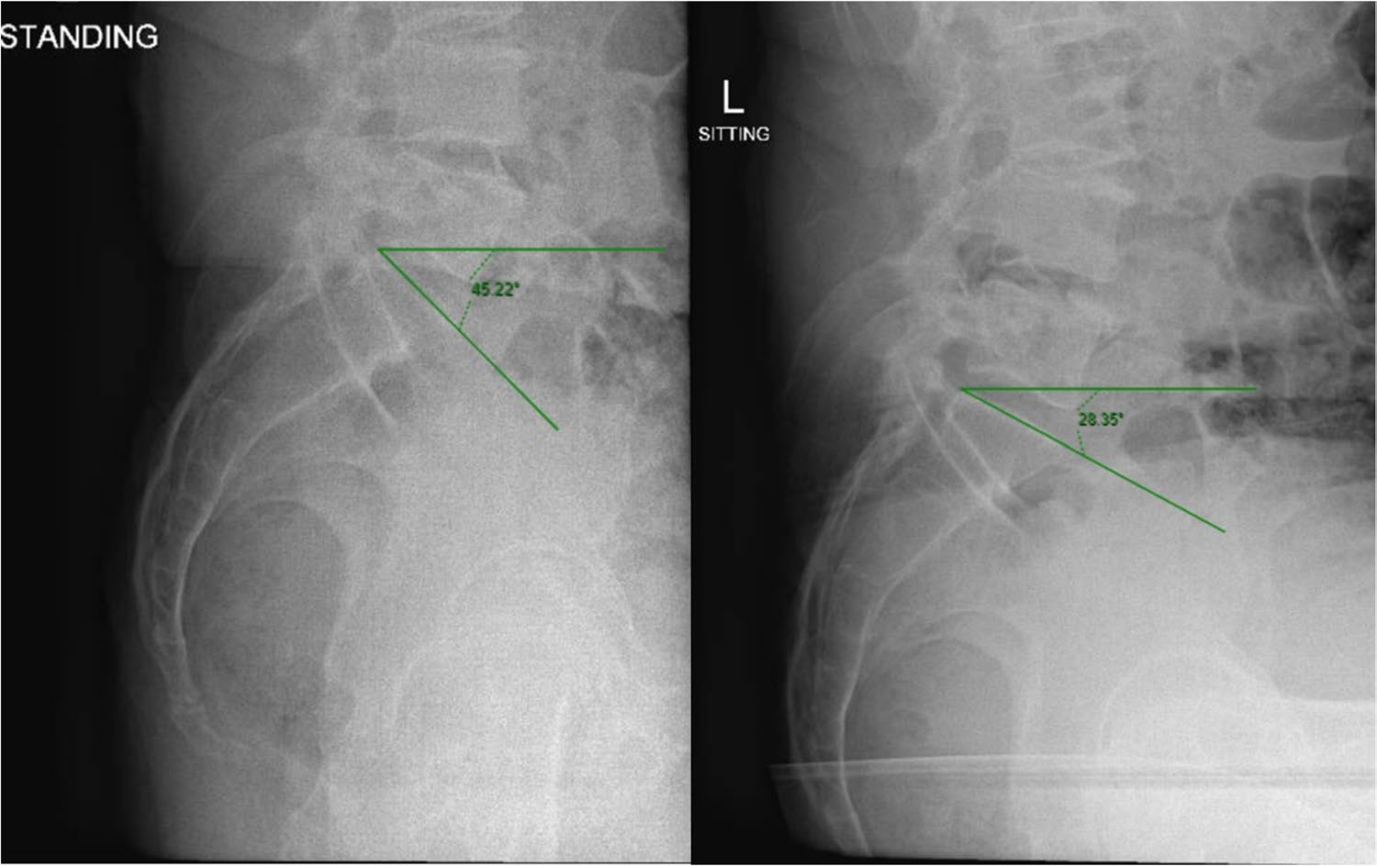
Lateral pelvis plain radiographs demonstrating sacral slope measurements in standing (left) and sitting (right) positions
